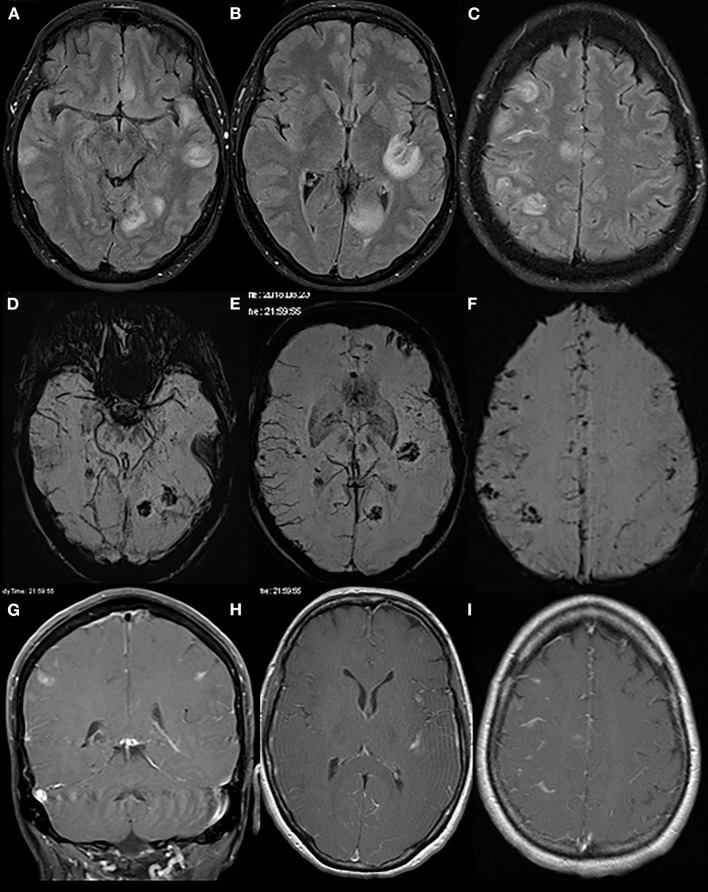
Initial brain MRI. Brain MRI displays multiple round/ovoid cortico-subcortical lesions with surrounding edema (T2-FLAIR sequences: A–C). Some lesions had a mainly central hemorrhagic component (susceptibility-weighted imaging, D–F). On contrast-enhanced T1 scans (G–I), there was partial lesional and leptomeningeal contrast enhancement.
